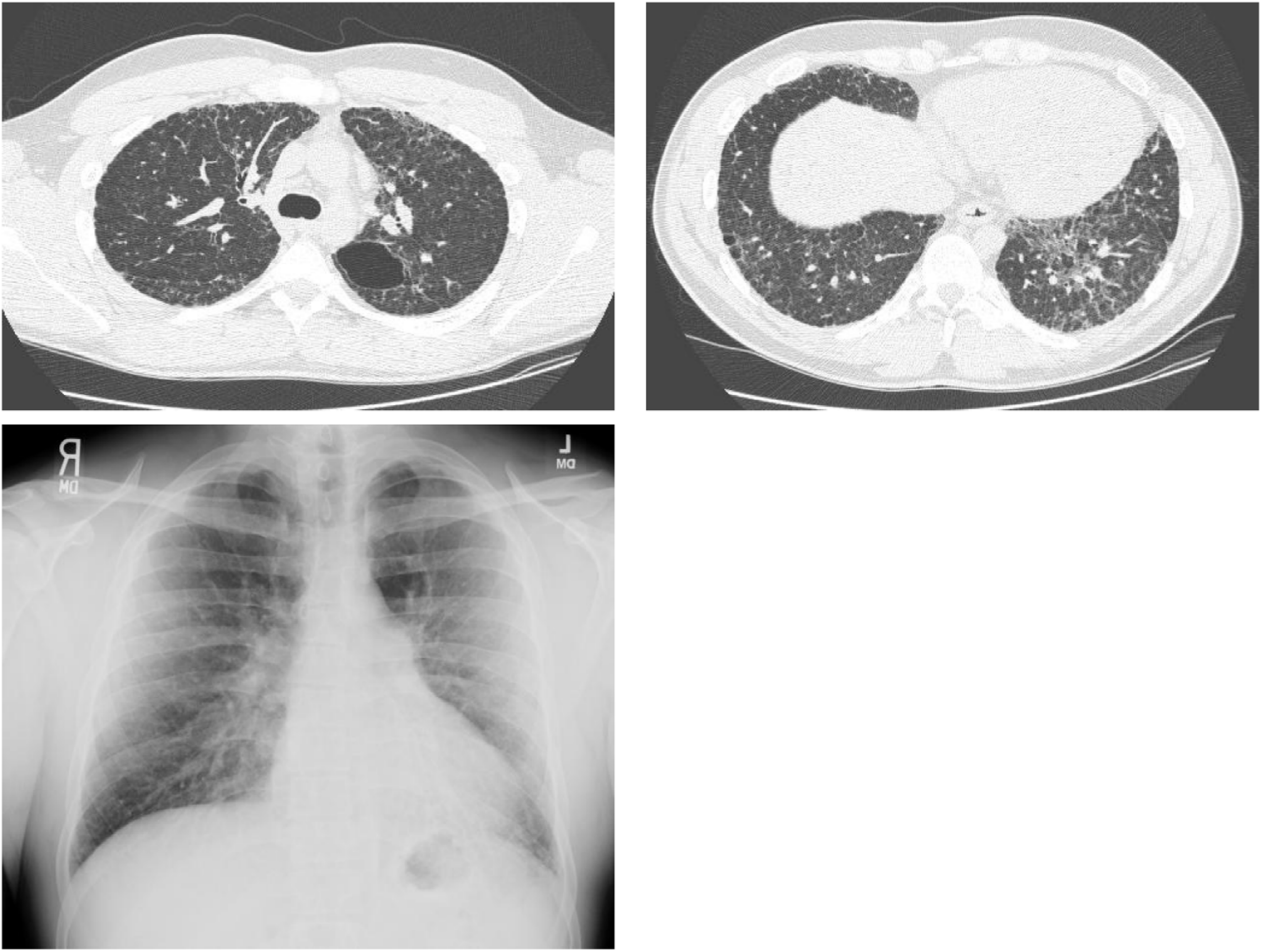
Chest CT and X-rays, patient age 27 years.
